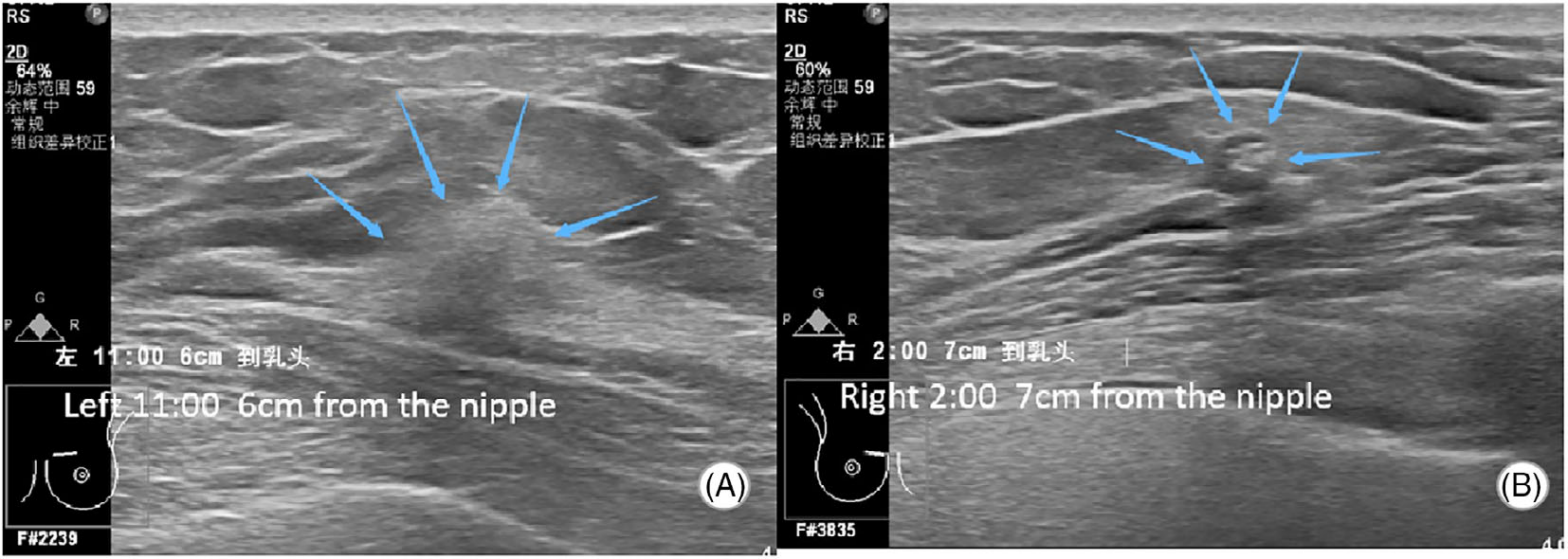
Ultrasonic findings of the bilateral breast lesions by AI‐breast US. (A) A heterogeneous hypoechoic lesion detected in the left breast in the 10‐ to 11‐o'clock position 6 cm away from the nipple. (B) A nodule with calcifications within the mass detected in the right breast in the 2‐o'clock position 7 cm away from the nipple
